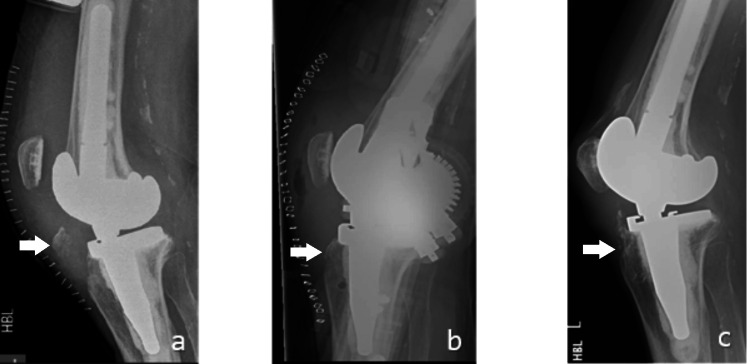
Serial lateral knee X-rays showing fracture tibial tuberosity (a), postoperative day 1 after fixation showing the successful reduction of the fracture (b), and postoperative after 18 month showing minimal proximal migration and good callus formation (c) Serial lateral knee radiographs: preoperative image showing tibial tuberosity fracture (arrow indicates fractured tuberosity) (a), postoperative day 1 image showing reduction and fixation (arrow indicates the fixation construct) (b), and 18-month postoperative image showing minimal proximal migration and good callus formation (arrow indicates united fracture) (c).
